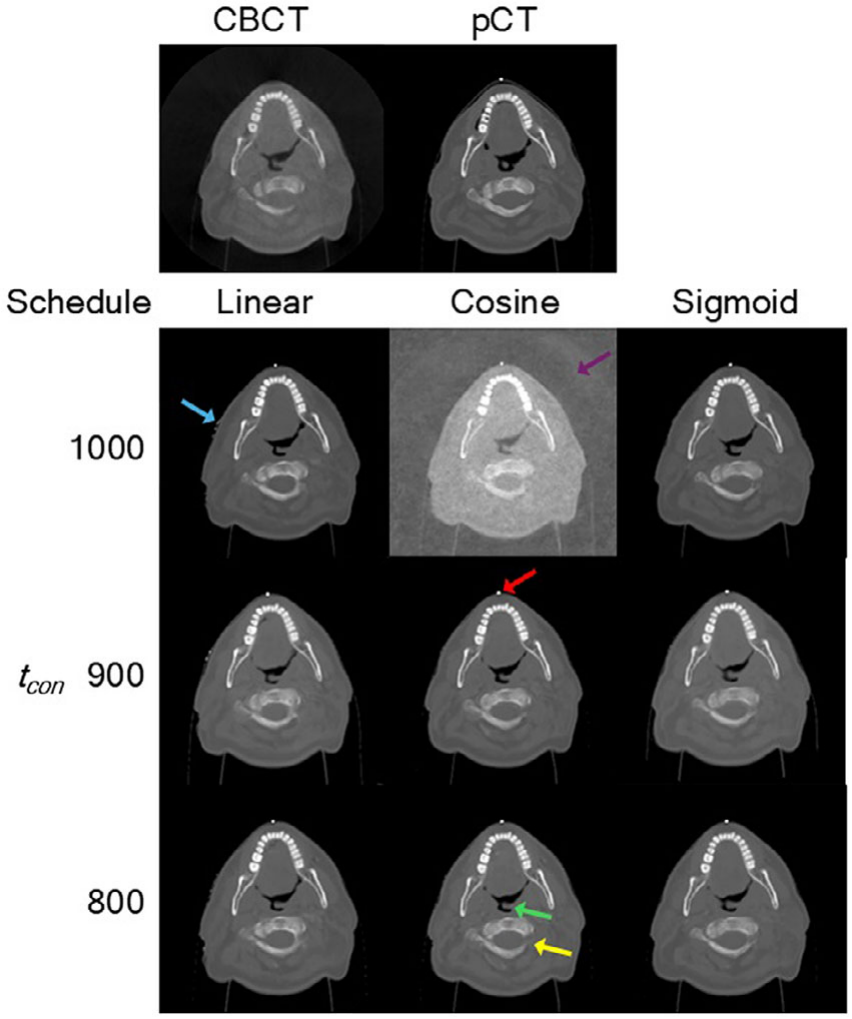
Synthetic CT images generated from different noise schedules and *t_cos_
